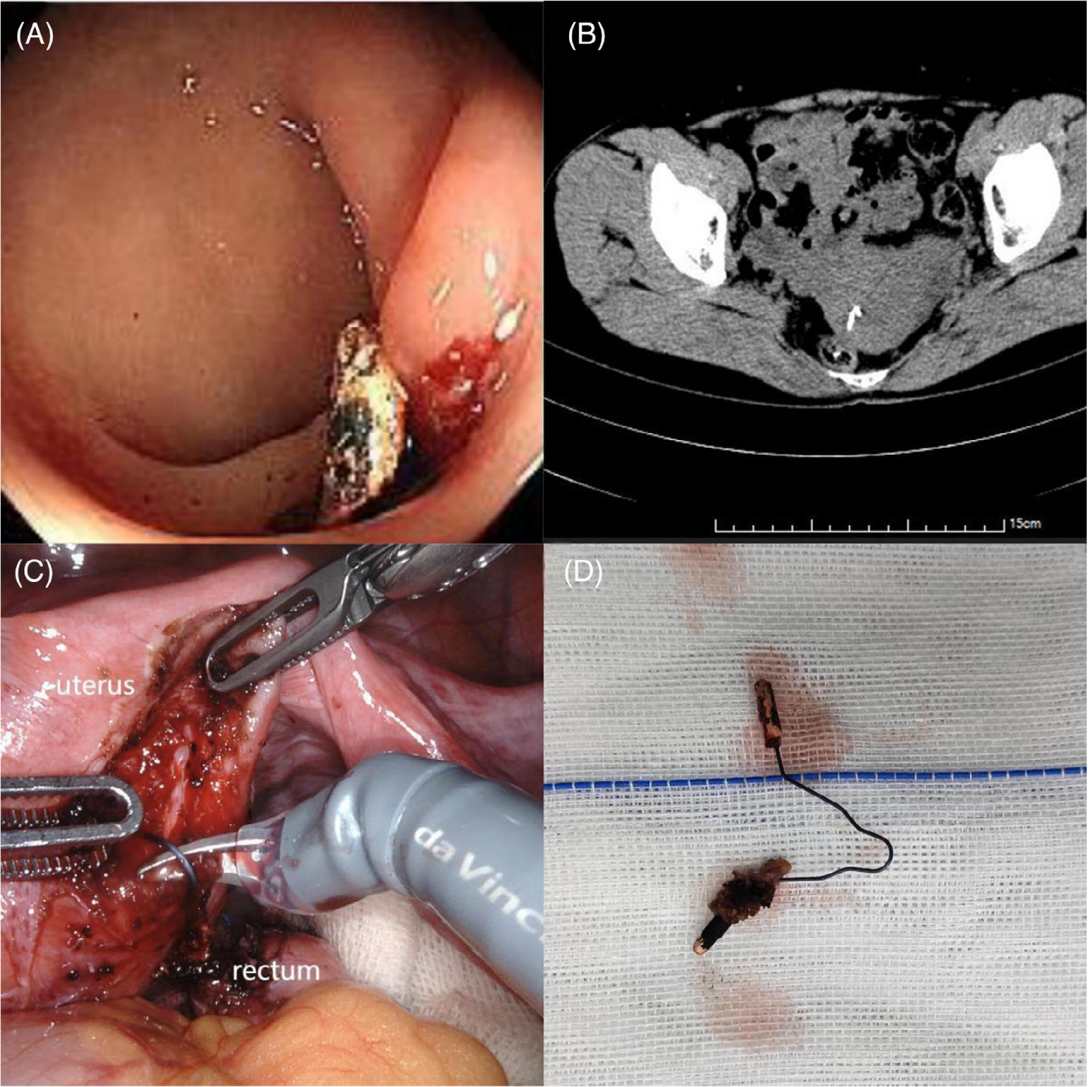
(A) Enteroscopy revealed a hard, immobile metallic rectal foreign body. (B) Abdominal CT showed a high density metal artifact at the right rear of the uterus, some of which entered the adjacent rectal cavity. (C) Robot‐assisted laparoscopy for rectal foreign body removal. (D) The foreign object was a “V” IUD.
